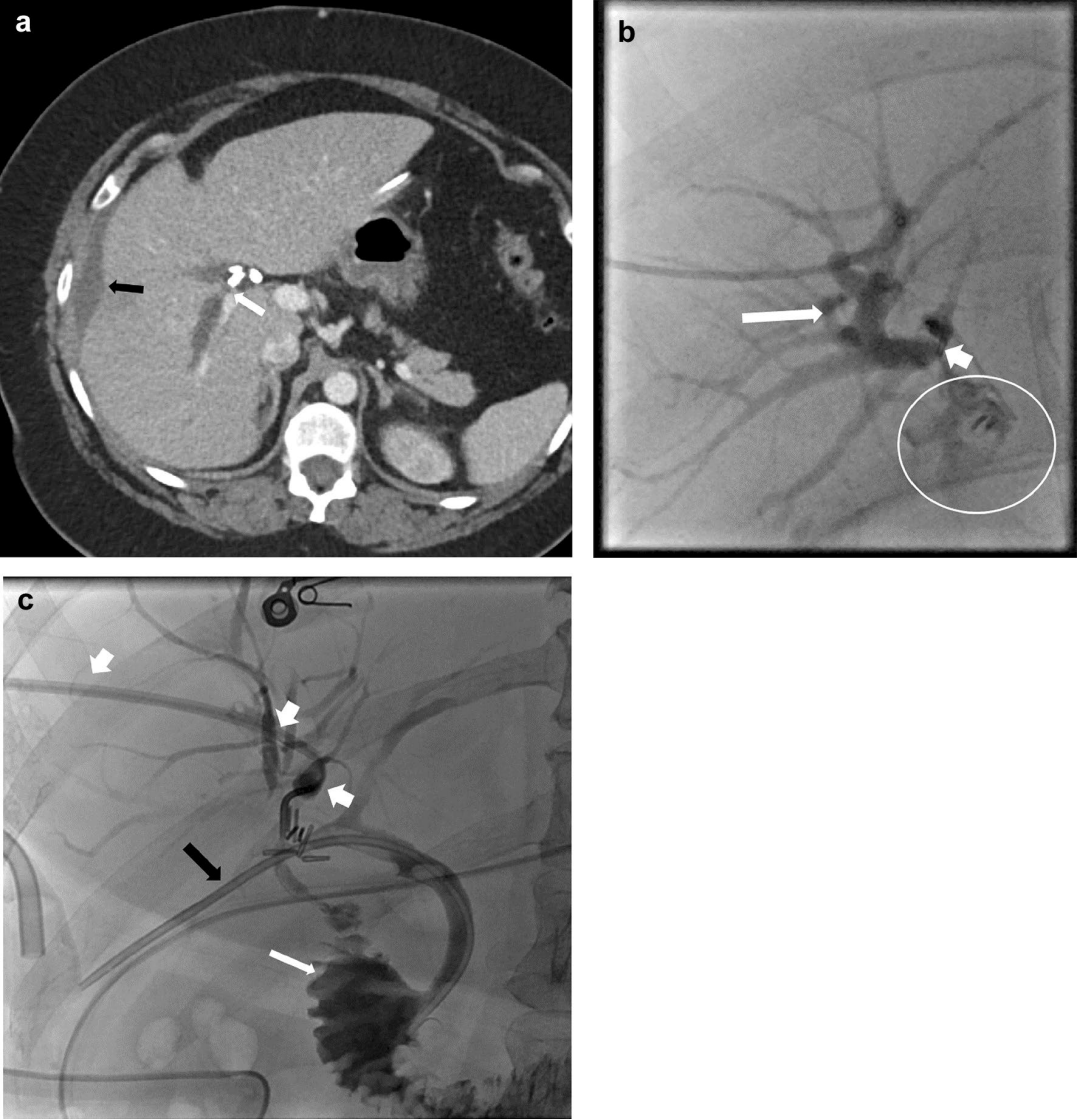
A 46-year-old female with right posterior hepatic duct injury, bile leak, and biliary obstruction involving the posterior segments of the right hepatic lobe. a axial CECT image demonstrating a biliary dilatation involving the right posterior hepatic lobe with abrupt narrowing adjacent to a surgical clip in the hepatic hilum (white arrow). Perihepatic fluid collection is also demonstrated (black arrow). MRI with Eovist contrast and MRCP also confirmed biliary dilatation in the right posterior hepatic lobe and leak of Eovist into the perihepatic collection (not shown). b PTC image demonstrating intrahepatic biliary dilatation (long white arrow) upstream to a surgical clip (short white arrow) and associated bile leak (white circle). c PTC post placement of internal/external bile drain (short white arrows) and recanalization of the occluded right posterior duct demonstrates the passage of the contrast into the duodenum (long white arrow). A common bile duct stent is also seen (black arrow)
